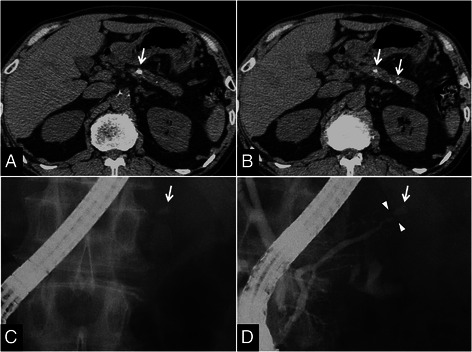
CT and ERCP findings in a 66-year-old man whose pancreatic stone was treated with ESWL to preserve pancreatic function. (A), (B) CT before ESWL showing the pancreatic stone and pancreatic atrophy (arrows). (C), (D) ERCP before ESWL identifying the obstructing X-ray-positive stone in the MPD (arrows) and pancreatic duct stenosis proximal to the pancreatic calculus (arrowheads). Pre-pancreatograpy (C) and post-pancreatography (D) images.
